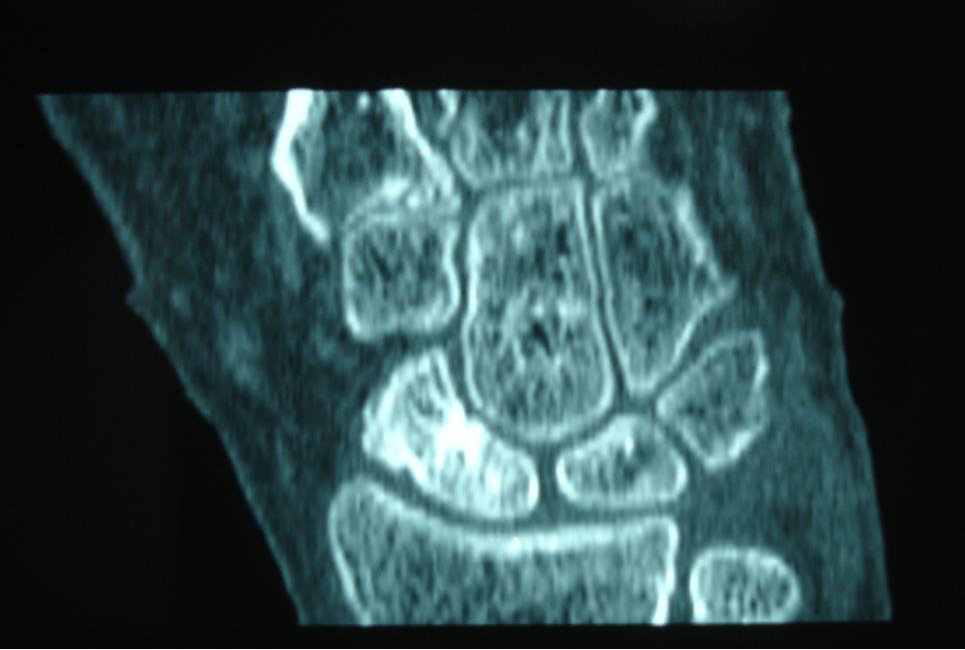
A postoperative CT scan of the healed SNU in patient number 3.
